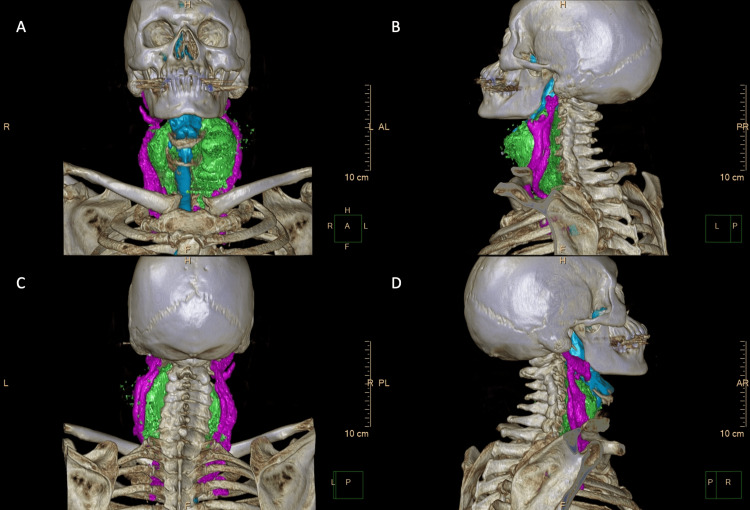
Three-dimensional CT reconstruction of liposarcoma. Anterior view (A), left lateral view (B), posterior view (C), and right lateral view (D) showing tumor (in green), airway structures (in blue), and vasculature (in pink).
